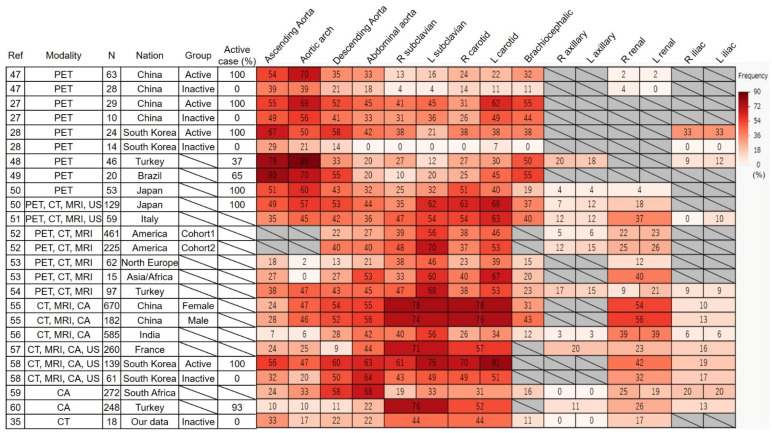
The frequency of arterial territory in Takayasu arteritis detected by several modalities in the literature is summarized as a heat map. Values are presented as percentages (%), with darker red indicating higher values. Abbreviations: CA, conventional angiography; CT, computed tomography; MRI, magnetic resonance imaging; PET, positron emission tomography; US, ultrasound.
